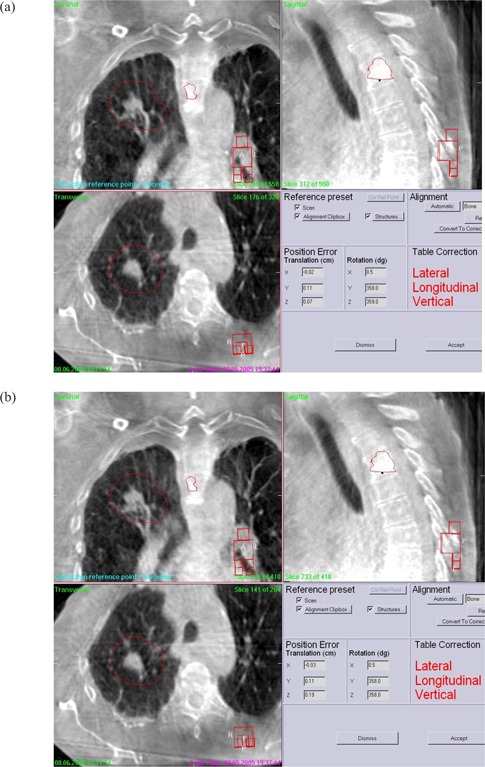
(a) High‐ and (b) medium‐quality reconstructed cone‐beam computed tomography images of lung and spine area. Both images clearly show lung mass and spinal bones. No easily observable significant difference is seen between the reconstructions.
